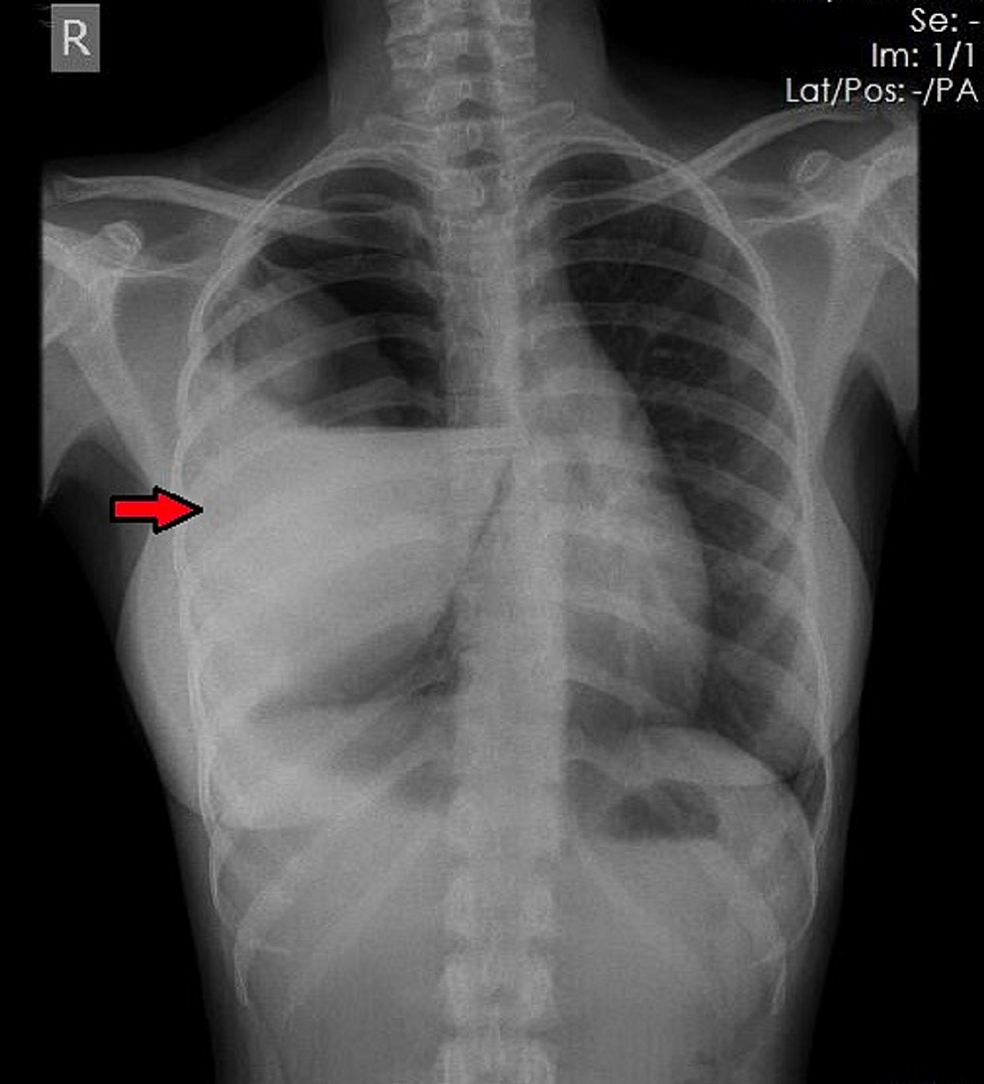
Preoperative chest x-ray showing a right hydropneumothorax
